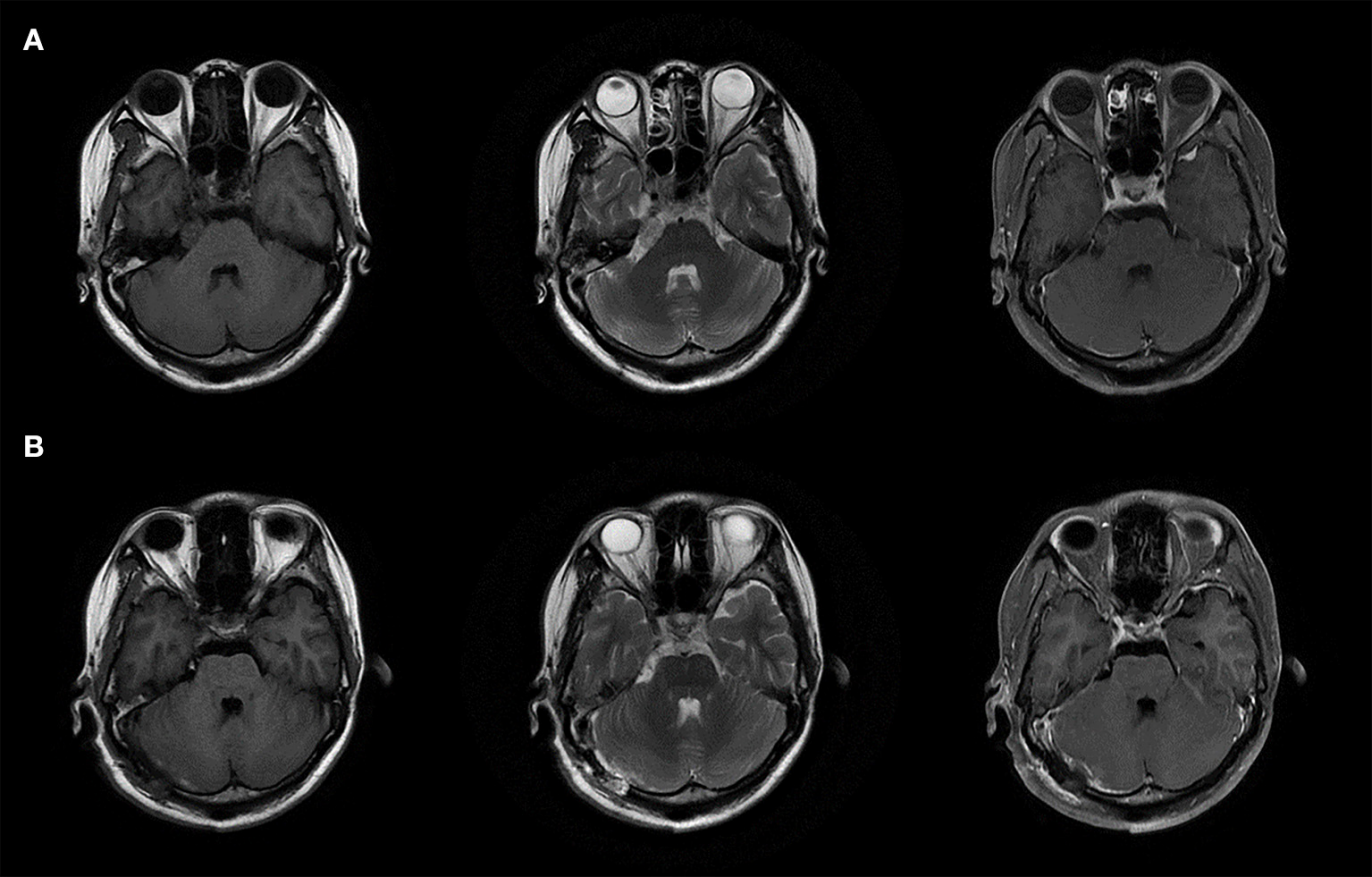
MRI images of the lesion. (A) Preoperative brain MRI images. (B) Postoperative brain MRI images.
